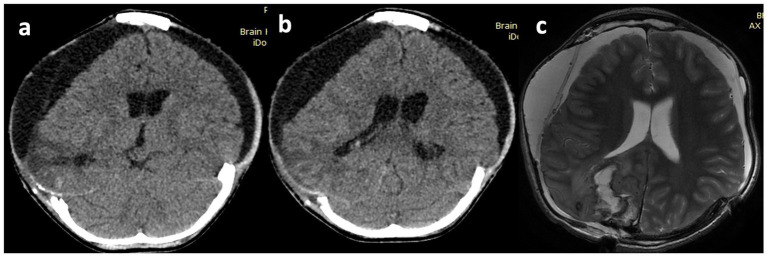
Traumatic bilateral subdural hygromas on: (a,b) head CT scan and (c) T2 weighted image brain MRI of a patient undergoing decompressive craniectomy for severe traumatic brain injury, 1 month ago.
